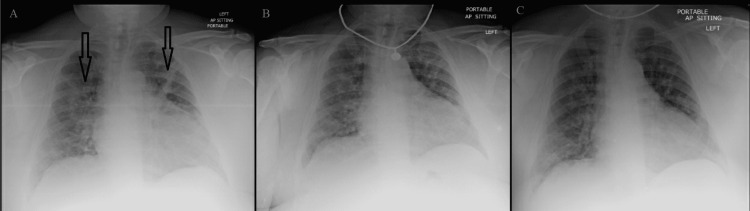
Twin A: chest X-ray on day one (A), day three (B), and day five (C). Arrows depict patchy bilateral infiltrates.
